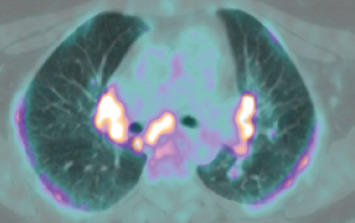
PET-CT body scan before discontinuation of pembrolizumab.
